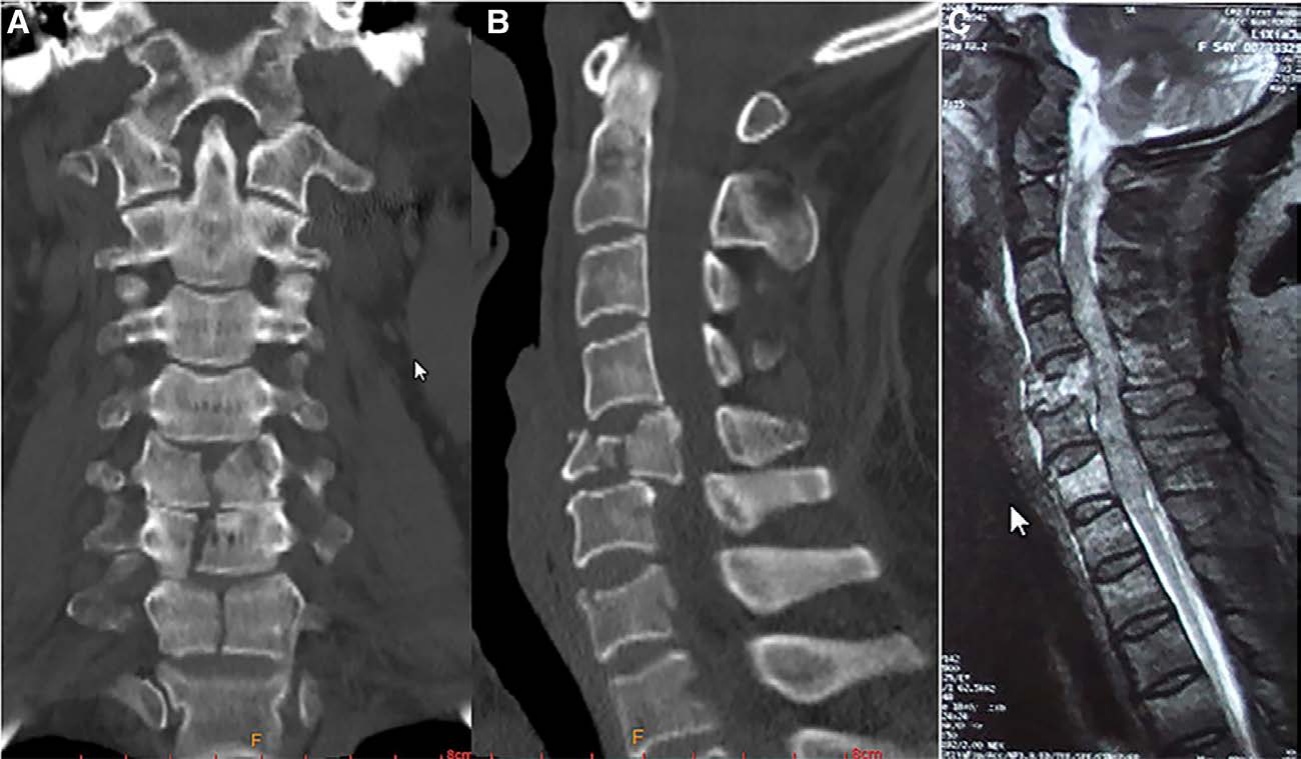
(a) Anteroposterior preoperative CT scan showing burst fracture with translational injury of C5 and longitudinal split fracture of C6 and C7, (b) lateral preoperative CT scan, and (c) lateral preoperative MRI showing a spinal cord edema extending from the epicenter of the injury up to C3 and down to C7.
